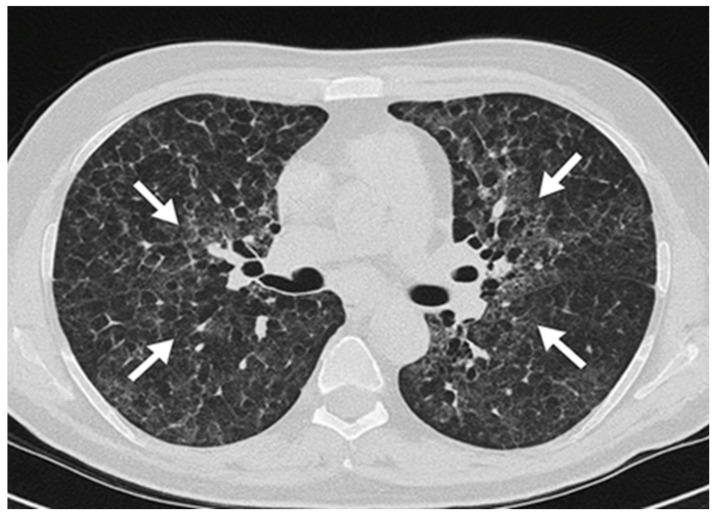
Expiratory HRCT demonstrating areas of air trapping (arrows), indicative of small airway involvement consistent with chronic HP.
